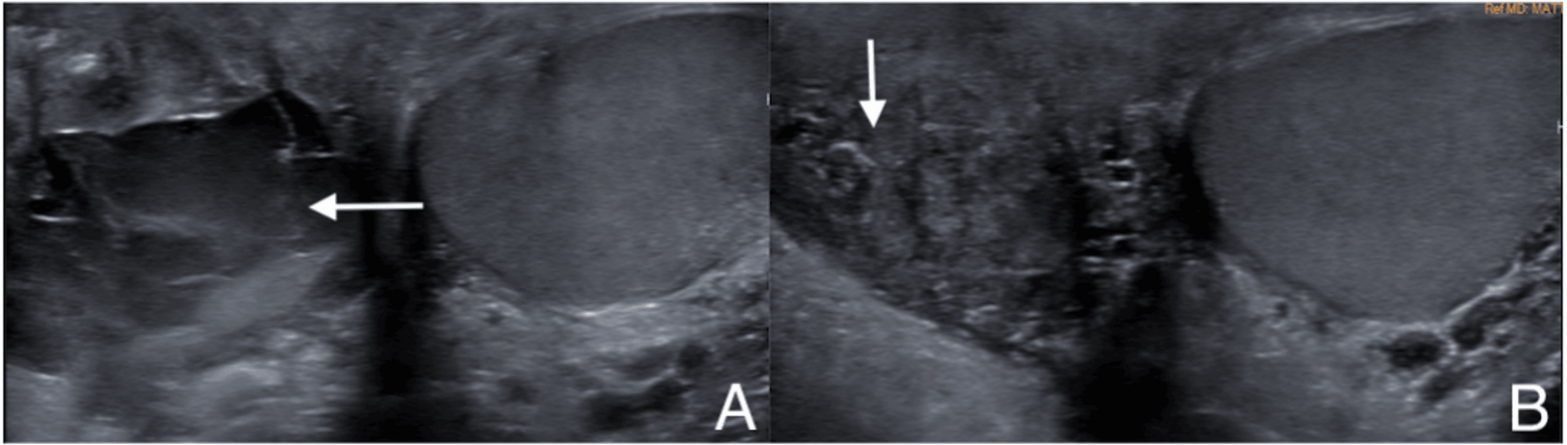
Post-right radical orchiectomy scrotal sonogram demonstrating complex fluid collection of 5.2 x 2.5 x 4.2 cm in right scrotal potential space
